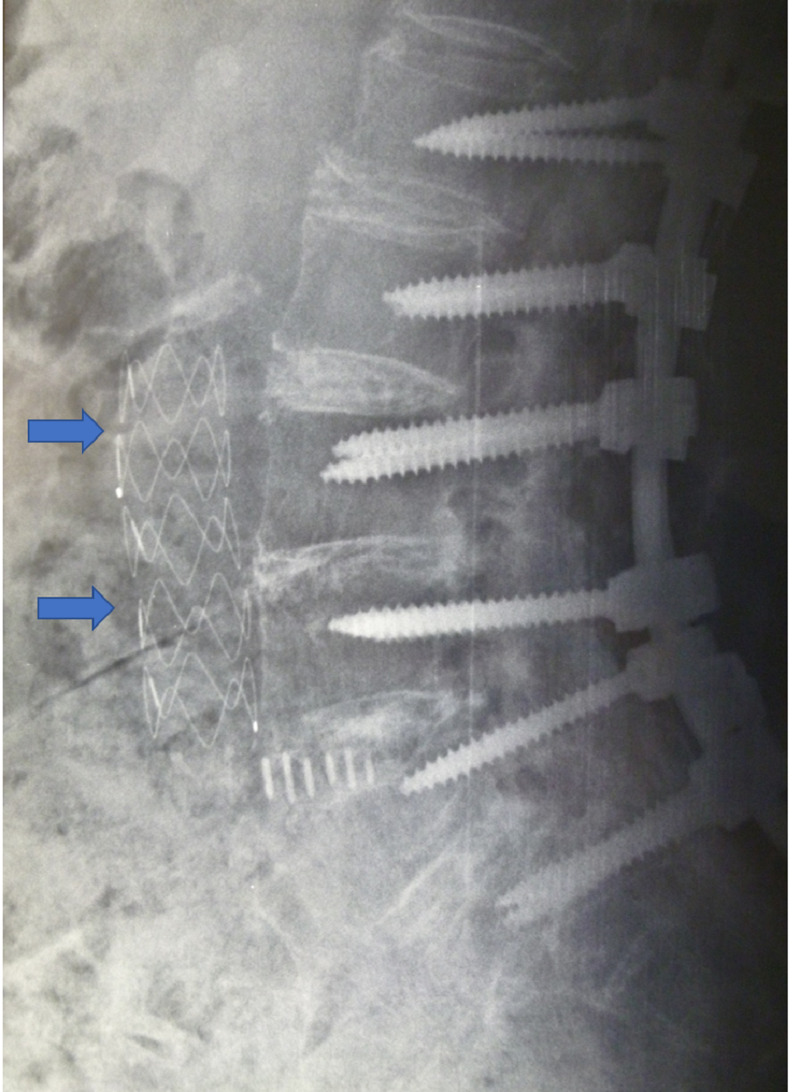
Lateral X-ray of the lumbar spine (sitting) on admission showing the instrumented lumbar spine. Intraluminal endovascular abdominal aorta mesh stent in situ (arrows).
